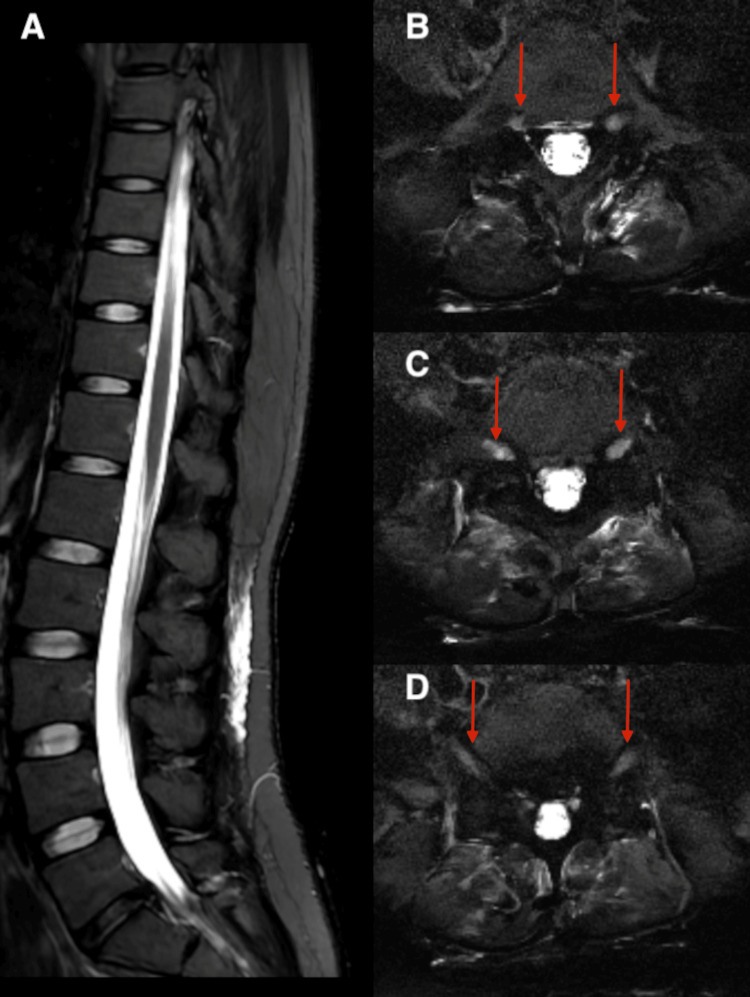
Short tau inversion recovery image of thoracolumbar spine MRI: (A) sagittal view and (B-D) serial axial view at the L5/S1 level No structural abnormalities were observed in the sagittal view. (B-D) The images show bilateral enlargement of the nerve roots (red arrows).
